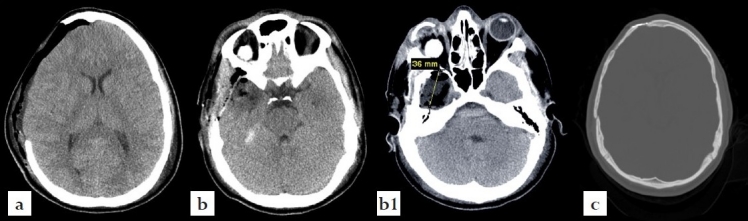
Postoperative CT scans of the brain (a) post-hemicraniectomy, (b) temporal lobectomy, and (c) after second stage autologous cranioplasty.
